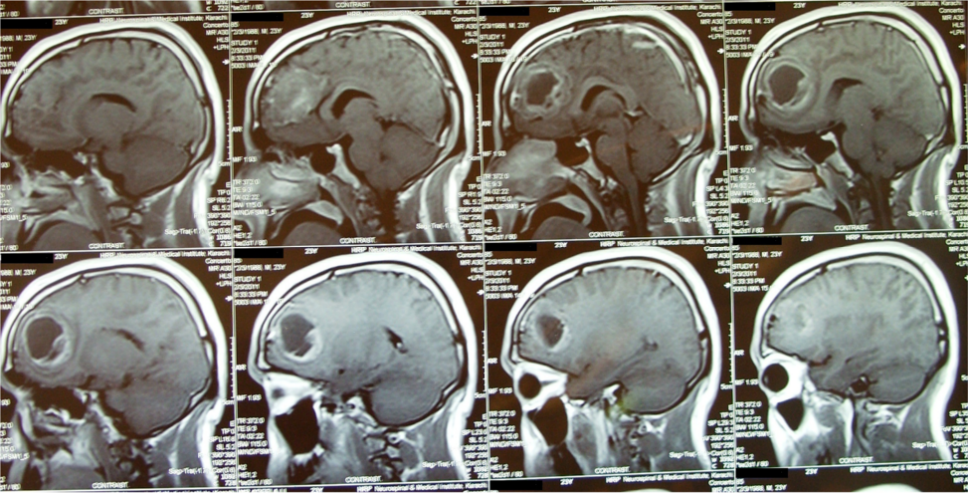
Sagittal view of T-1 weighted magnetic resonance images with contrast revealing chondroma. Sagittal magnetic resonance images of the brain conducted 3 days before surgery.
